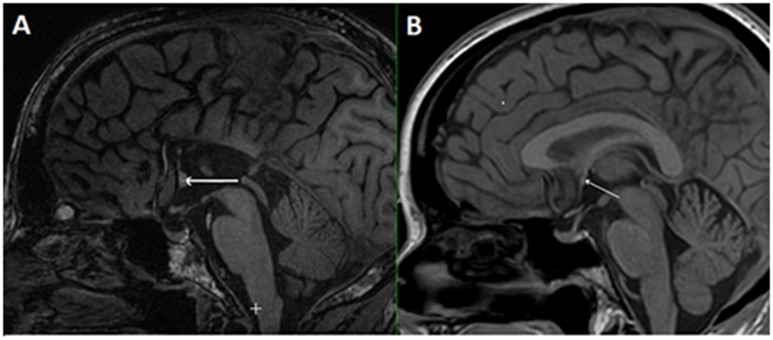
Mid-sagittal plane MRI section of the individual with callosal agenesis (A). The arrow indicates the enlarged anterior commissure (diameter 5.2 mm) as compared to a control individual (B) with a corpus callosum and a normal anterior commissure with a diameter of 1.8 mm (arrow).
